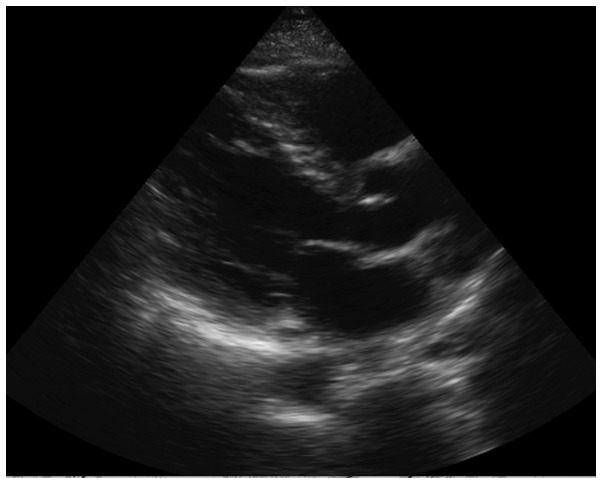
Echocardiography at the one-year follow-up shows no sign of recurrence.
